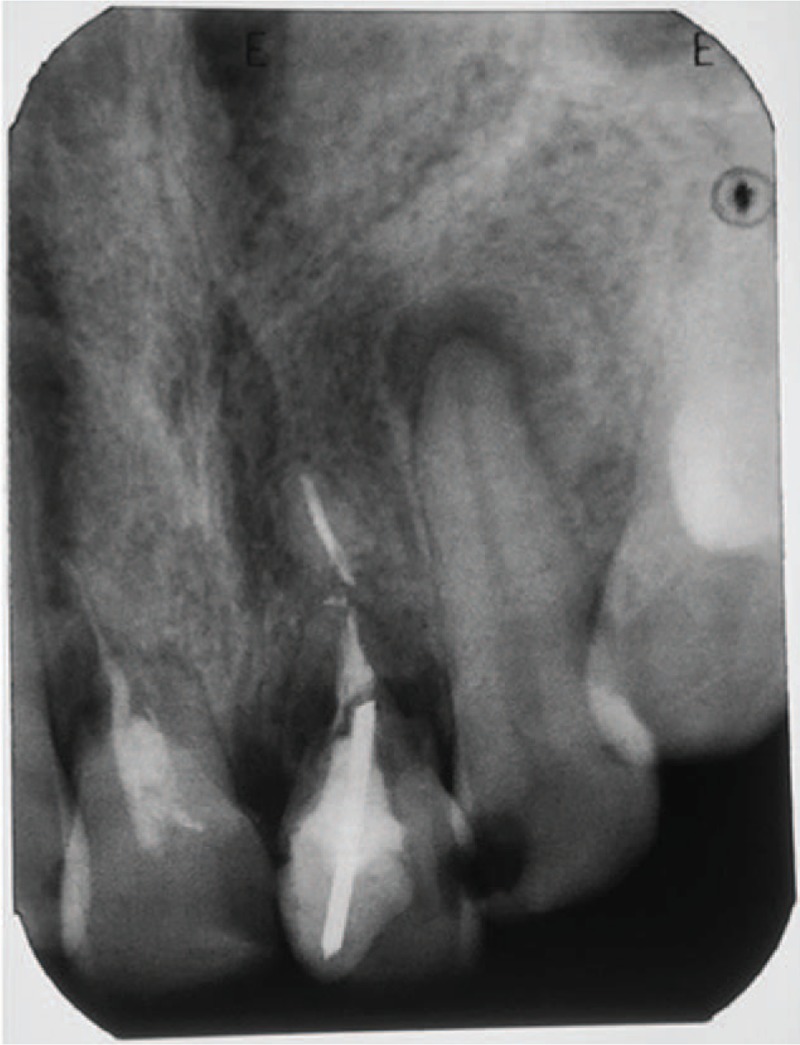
Roots of the upper central incisors showing external resorption. The right incisor was resorbed along with the root surface. In the left center incisor, resorbed areas were observed on the distal and mesial profiles of the tooth in the cervical and middle thirds. No radiolucency seen in the periapical regions.
